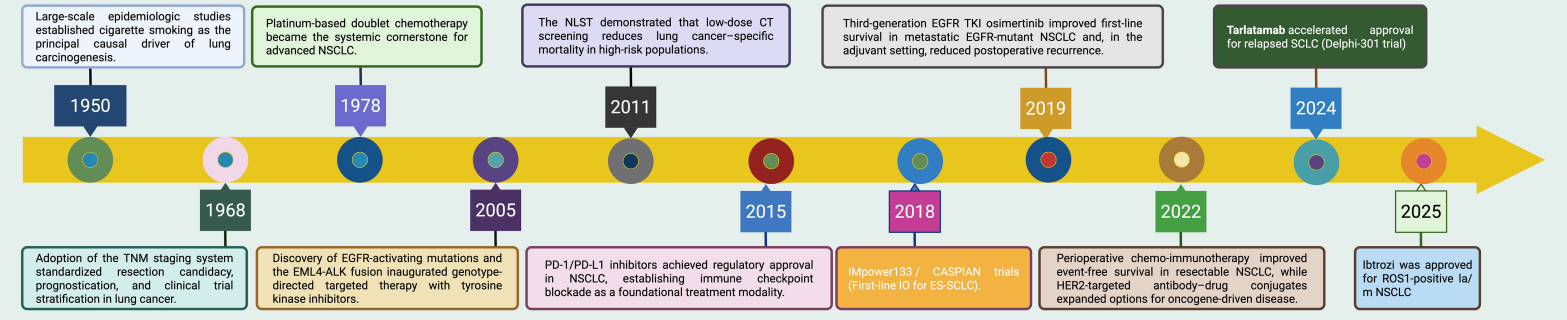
Evolution of lung cancer management and immunotherapy milestones (1950–2025). Landmark advances that have shaped lung cancer prevention, diagnosis, and systemic therapy from 1950 to 2025. Key inflection points include: (i) recognition of cigarette smoking as the principal causal driver of lung carcinogenesis (1950), catalyzing prevention policies; (ii) adoption of the TNM staging system to standardize resection candidacy, prognostication, and clinical-trial stratification (1968); (iii) establishment of platinum-based doublet chemotherapy as the systemic backbone for advanced NSCLC (1978); (iv) the NLST demonstration that low-dose CT screening reduces lung cancer–specific mortality in high-risk populations (2011); (v) discovery of EGFR-activating mutations and EML4-ALK fusion (2005), followed by third-generation EGFR TKI improving first-line survival and reducing postoperative recurrence (2019); (vi) regulatory approval of PD-1/PD-L1 inhibitors, establishing immune checkpoint blockade as a foundational treatment modality (2015); (vii) establishment of chemo-immunotherapy as the first-line standard for extensive-stage SCLC based on the IMpower133 and CASPIAN trials (2018); (viii) perioperative chemo-immunotherapy improving event-free survival in resectable NSCLC and expansion of HER2-directed antibody–drug conjugates (2022); and (ix) accelerated approval of tarlatamab for relapsed SCLC (2024), followed by the approval of Ibtrozi for ROS1-positive NSCLC (2025). NSCLC, non-small cell lung cancer; CT, computed tomography; NLST, National Lung Screening Trial; EGFR, epidermal growth factor receptor; ALK, anaplastic lymphoma kinase; TKI, tyrosine-kinase inhibitor; PD-1/PD-L1, programmed cell death-1/ligand-1; ICI, immune checkpoint inhibitor; HER2, human epidermal growth factor receptor 2; ADC, antibody–drug conjugate; EFS, event-free survival.
